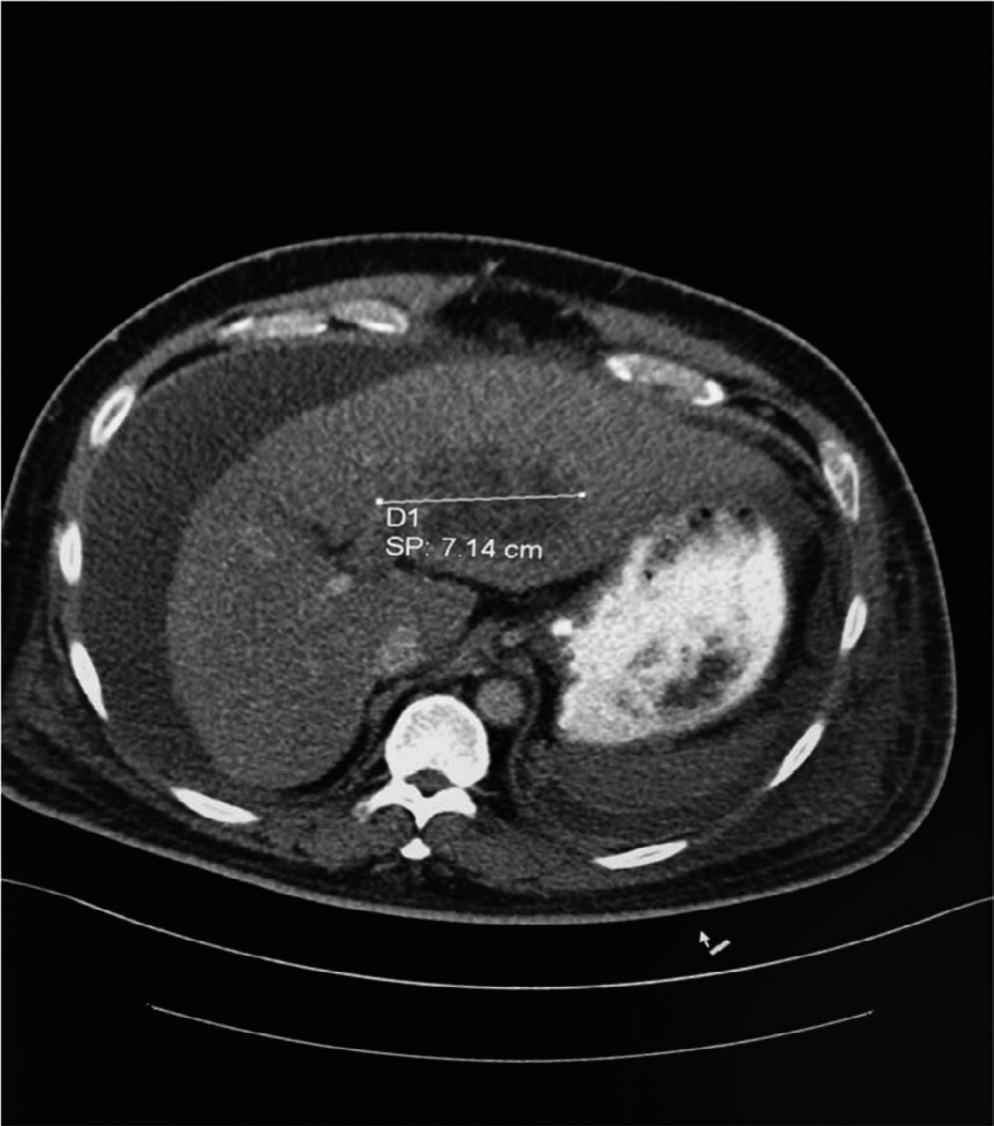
CT scan abdomen with contrast showed 7.14 cm multiloculated left liver lobe abscess
